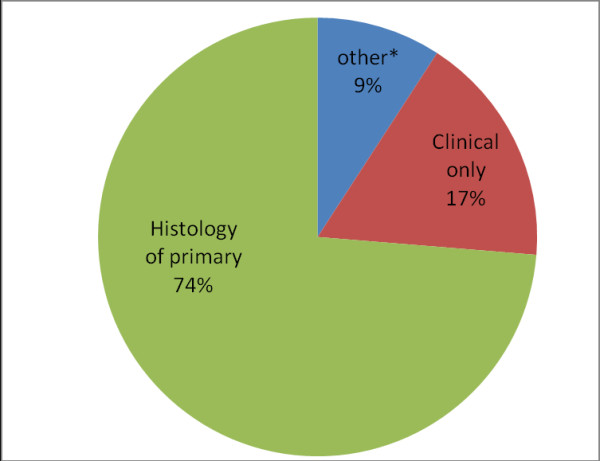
Basis of diagnosis for cancer cases in Kumasi, 2012. *includes haematological investigation, ultrasonography and tumour markers.
